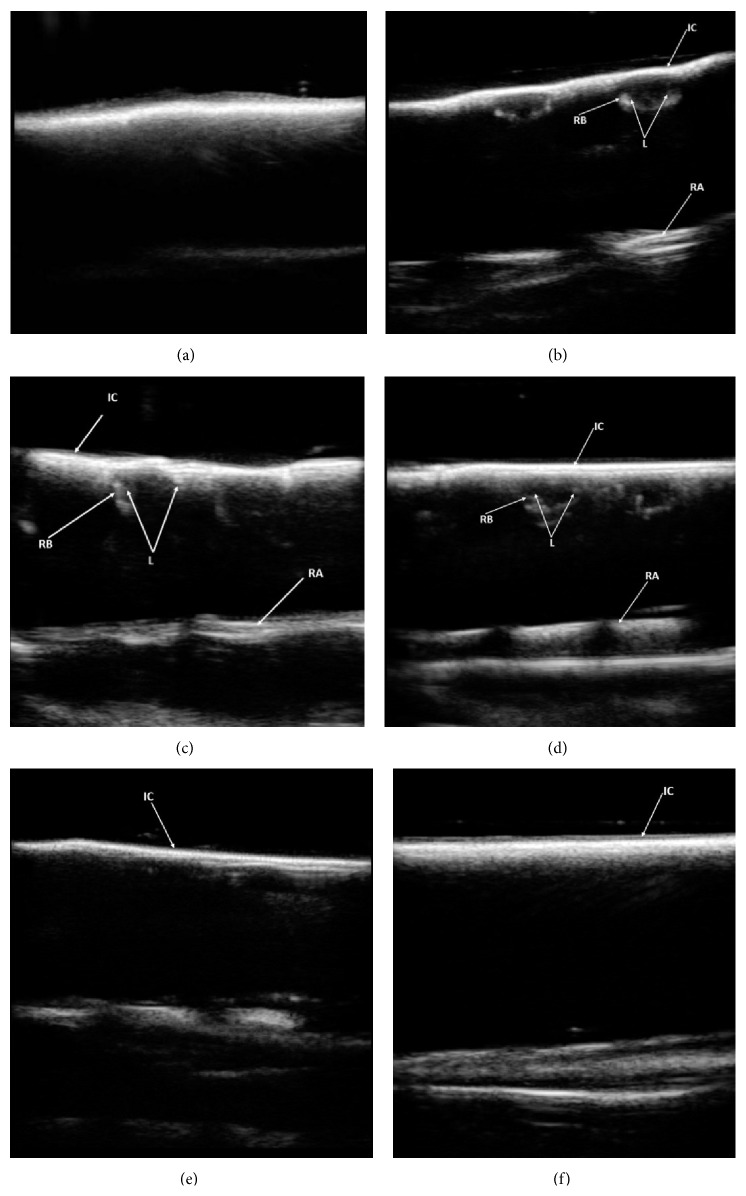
(a) Ultrasonogram of intact bone without any lesion. Ultrasonograms of sample: (b) number 1, (c) number 2, (d) number 11, (e) number 12, and (f) number 20. IC (intact cortical bone), RB (remaining bone between cavities), RA (reverberation artifact), and L (lesion).
